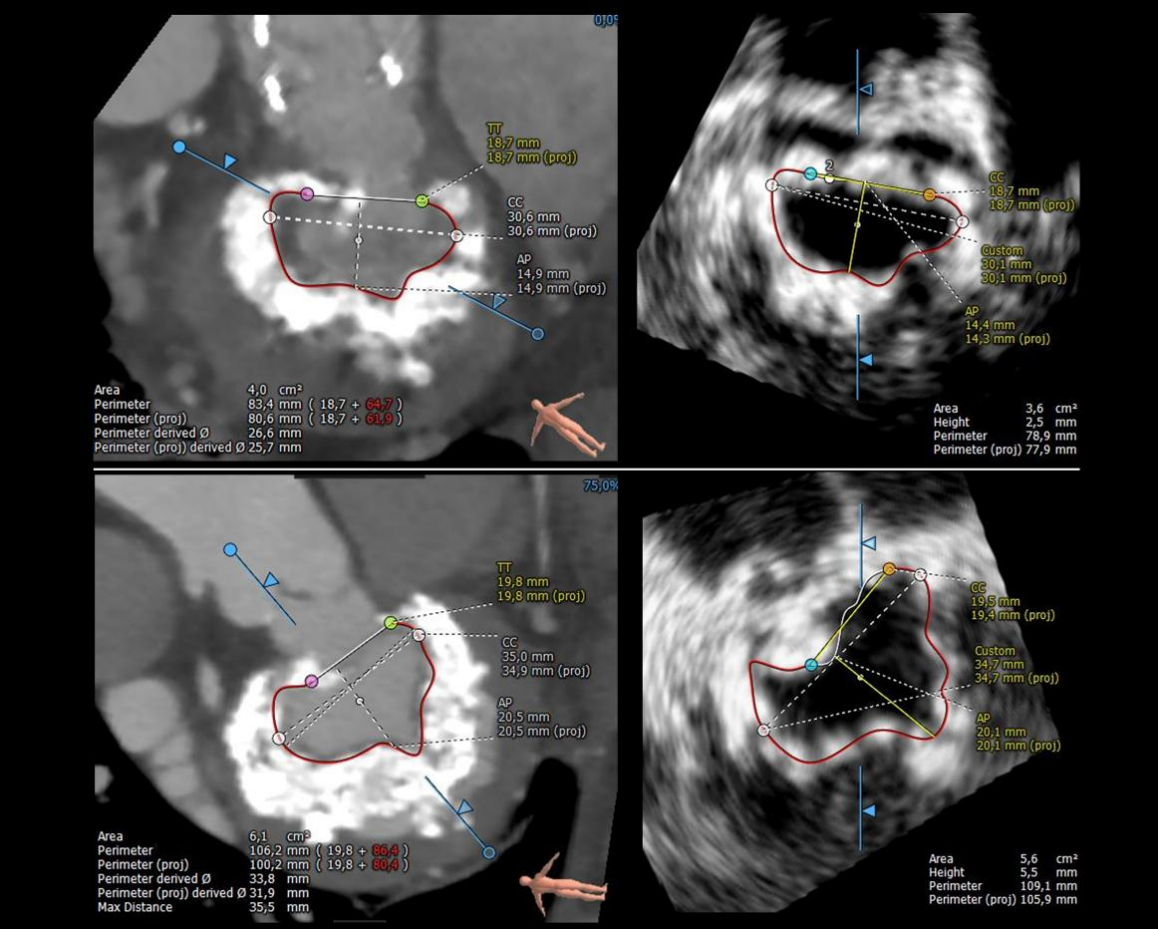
Valve in MAC: segmentation of the calcified mitral annulus by TOE and CT imaging using the D-shape method.
